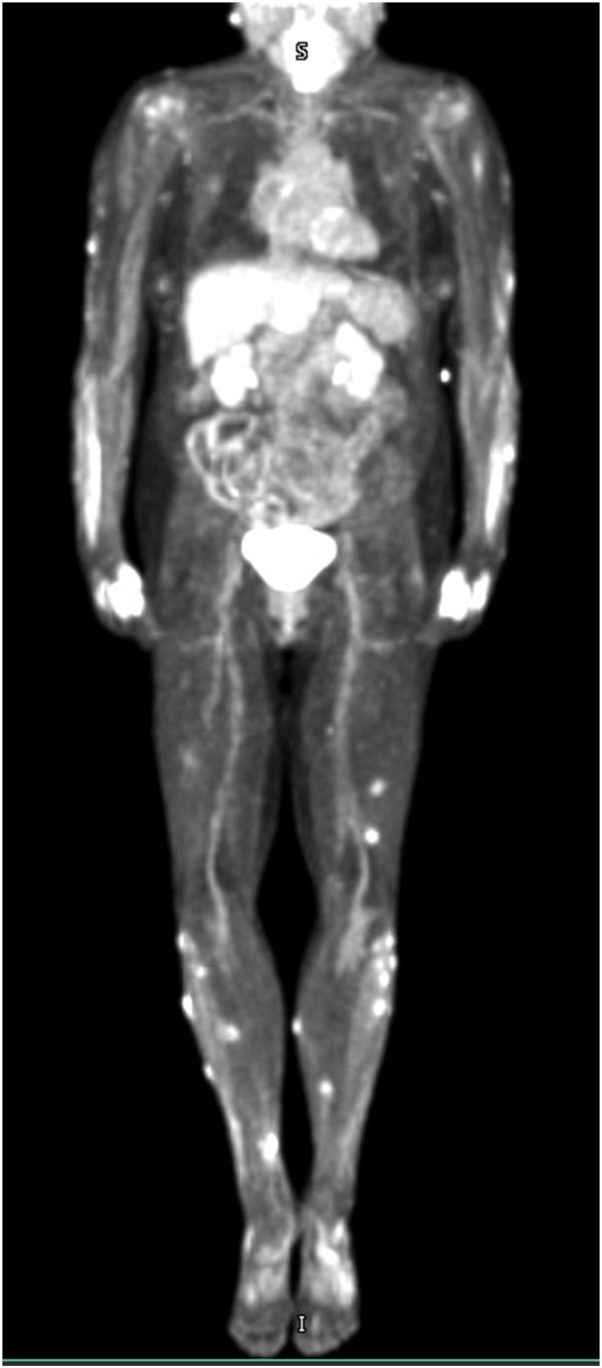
PET-CT body. Imaging showed diffuse hypermetabolic skin lesions concerning for malignancy, but no focal hypermetabolic activity in the brain, chest, abdomen, or pelvis to suggest internal metastasis. *PET-CT,* Positron emission tomography-computed tomography.
